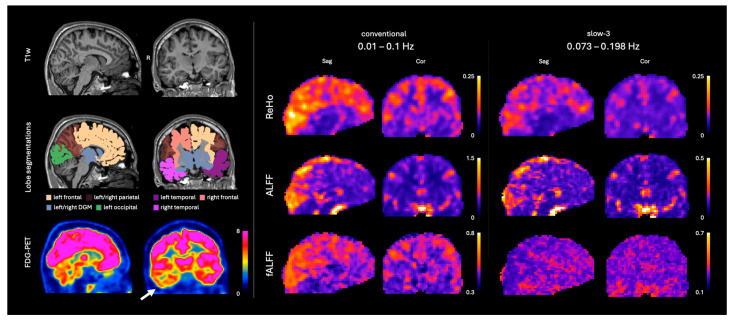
Overview of the utilized images and masks from a representative case (15 y male). The white arrow indicates the right-sided temporal lobe hypometabolism. T1w: T1-weighted image; FDG-PET: fluorodeoxyglucose positron emission tomography; ReHo: regional homogeneity; ALFF: amplitude of low-frequency fluctuations; fALFF: fractional ALFF; DGM: deep grey matter; Sag: sagittal view; Cor: coronal view.
